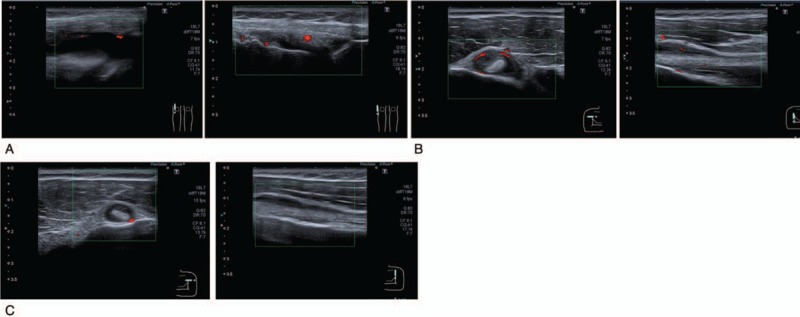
Musculoskeletal ultrasound (MSUS) findings after the initial treatment. MSUS findings when arthritis exacerbated with an increased level of C-reactive protein (CRP) (23.16 mg/dL), at September 2017. (A) Synovitis in the right knee joint and (B) tenosynovitis in the right bicep tendon exacerbated. (C) Tendon sheath thickening of the left biceps tendon newly appeared.
